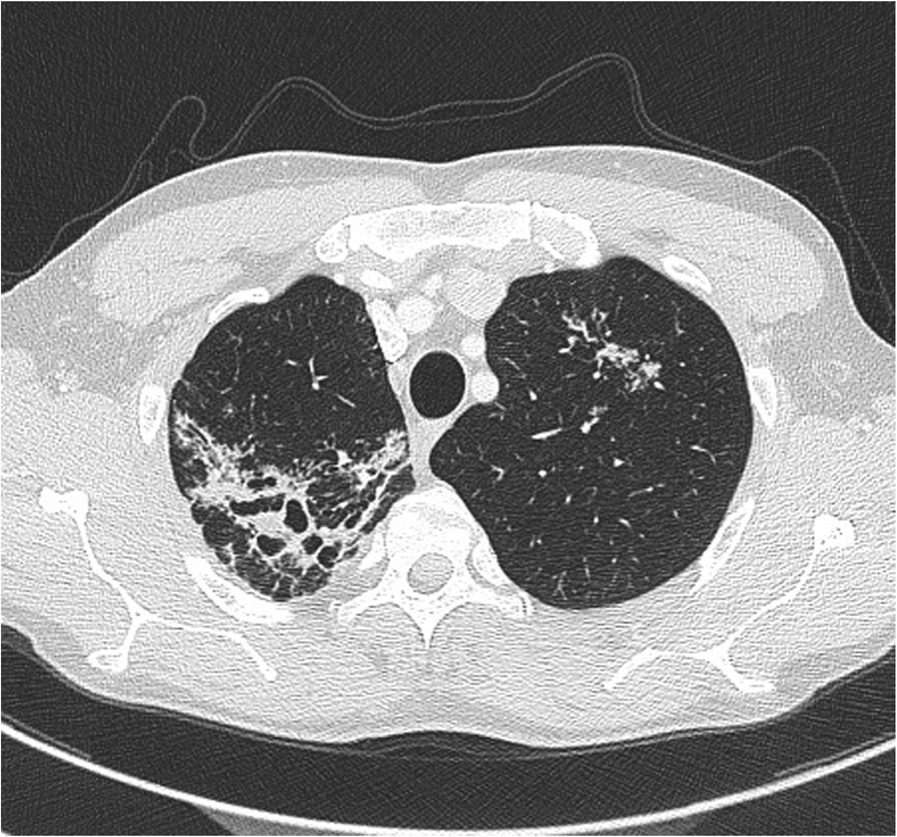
Thoracic computed tomographic scan showing a lesion suggestive of tuberculosis in the right apex and diffuse micronodular involvement.
